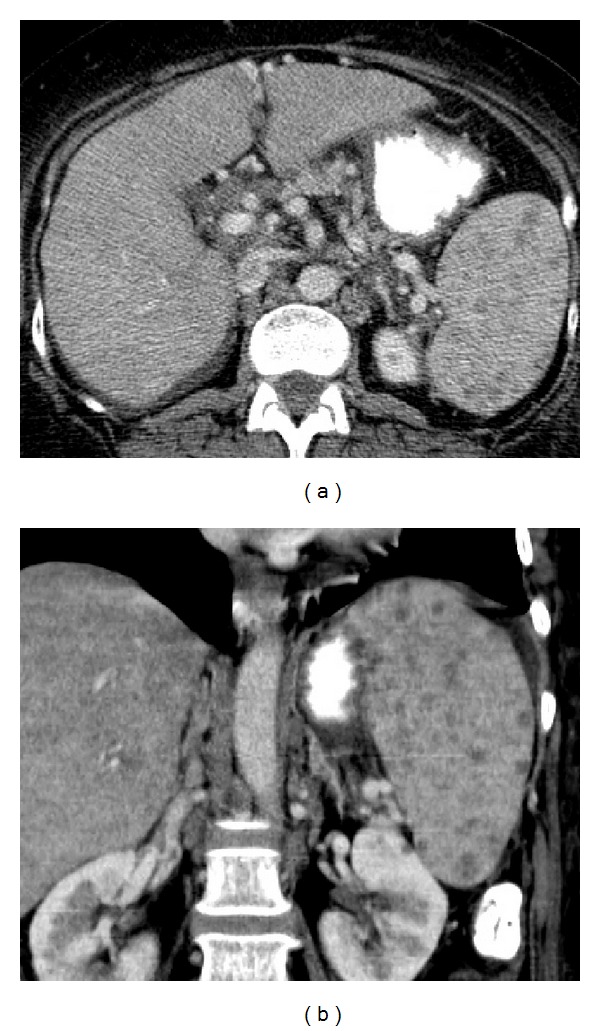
Gamna-Gandy bodies in a patient with portal hypertension. Axial (a) and coronal (b) contrast-enhanced CT images demonstrate an enlarged spleen with multiple low density foci representing hemosiderin deposition.
